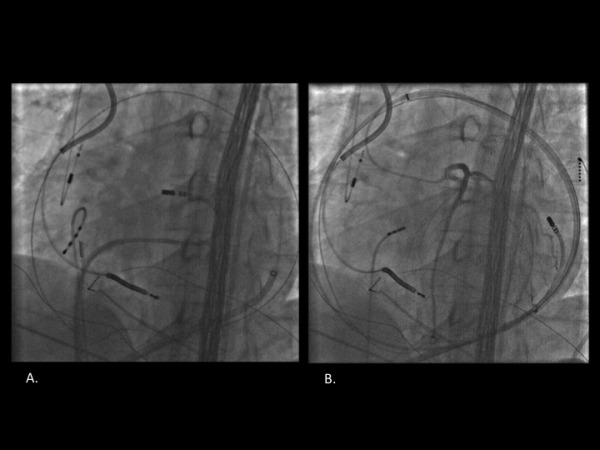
LAO fluoroscopic location of endocardial/proximal pace-map site (A) and radiographically distant epicardial/exit pace-map site (B).
